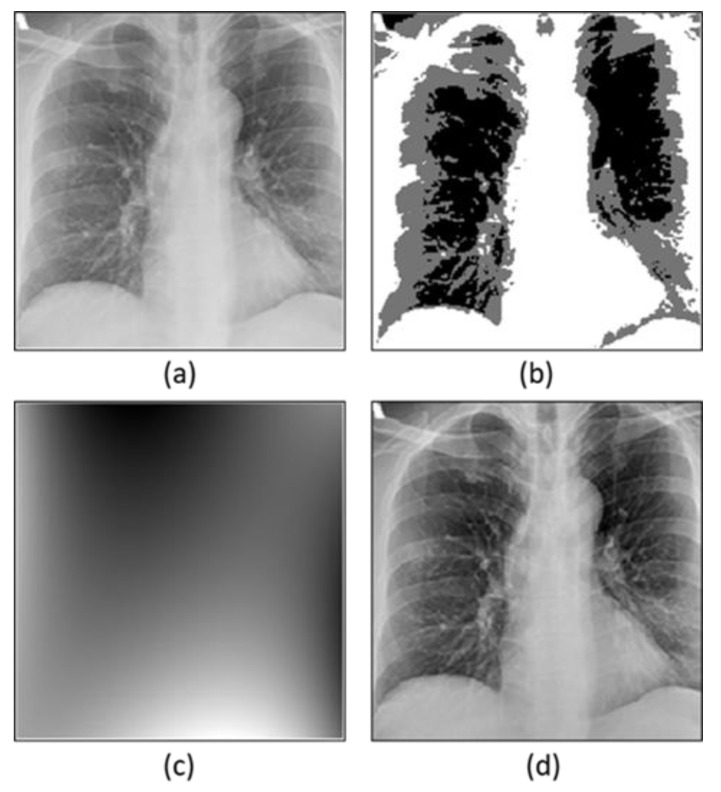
Application of bias field correction of the X-ray image. (a) Original image, (b) segmentation of inhomogeneous intensity, (c) estimated bias field image, (d) bias field correction image.
